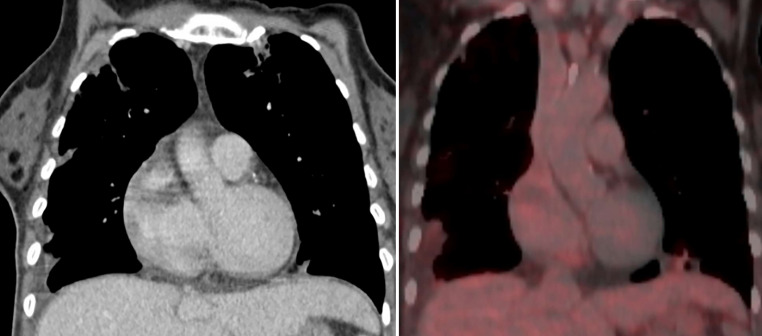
PET-CT (positron emission tomography-computed tomography) scan showing a 20-mm mass in the right ventricle
